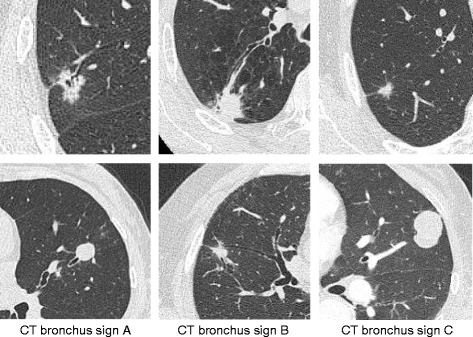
CT bronchus sign. According to TSCT (0.5-mm reconstruction), we categorized the CT bronchus sign in each case into three types a to c according to the relationship between the nearest bronchus and the target lesion. In type *A*, the responsible bronchus clearly reached the inside of the target lesion. In type *C*, no bronchus could be detected in relation to the lesion. When the CT findings could be categorized into neither type *A* nor *C*, the CT bronchus sign was categorized as type *B*
